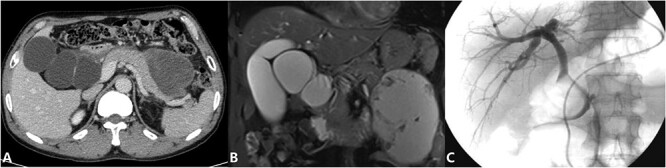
(Preoperative image) A: CT; B: MRCP; C: ERCP.
